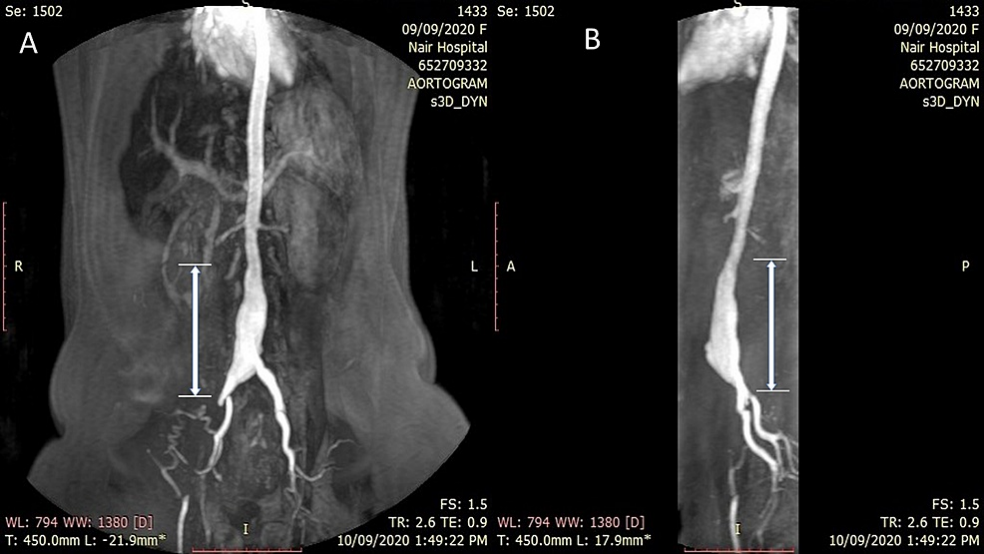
CT aortogram Coronal (A) and sagittal (B) section showing long segment (vertical extent-7.9 cm) fusiform dilatation of infrarenal part of the abdominal aorta
